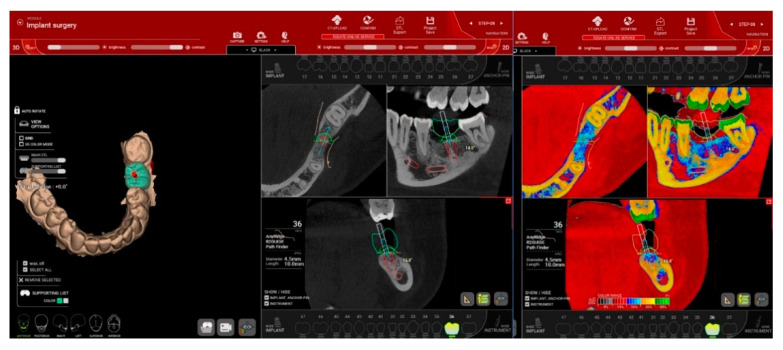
Planning implant directions, length and diameter in R2GATE software taking into consideration the final restoration and the available bone volume and quality. To facilitate bone quality assessment the “Digital Eye” option of the software (right) provides automatic conversion of CBCT gray scale in 5 basic colors, for bone density evaluation.
